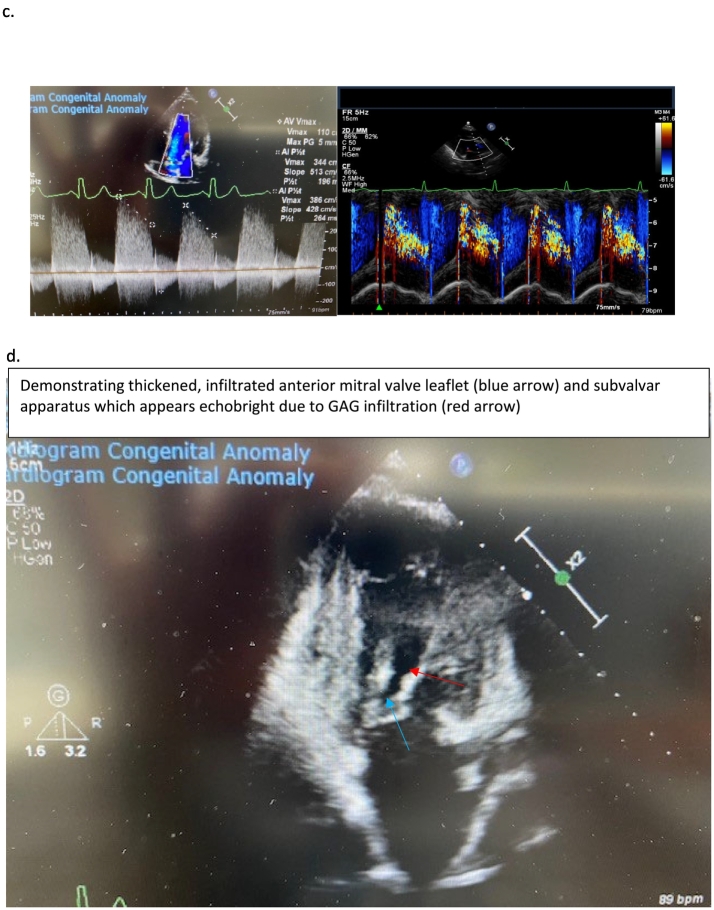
Echocardiogram features.
